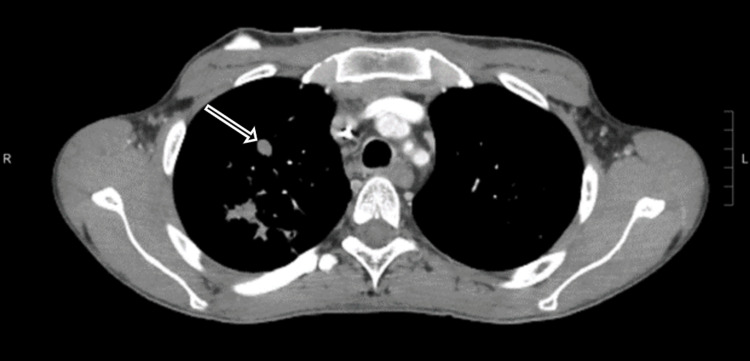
Soft-tissue window 1-mm transverse CT sections show a smoothly marginated 1 cm solid nodule (arrow) in the upper lobe of the right lung
